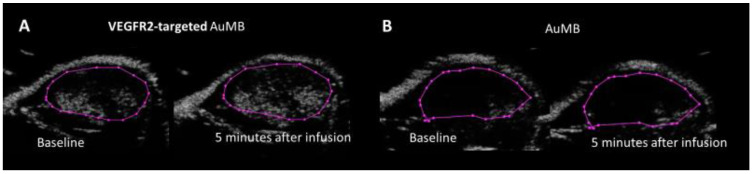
Representative harmonic B-mode ultrasonic images (20-MHz transducer) of xenograft tumors with VEGFR2-targeted AuMBs (A) or AuMBs (B) infused at baseline and 5 min later. The dynamic range was 50 dB with gain of −26 dB. The average enhancement in the pixel intensity was 7.84 vs. 0.27 a.u. (*p* < 0.05).
